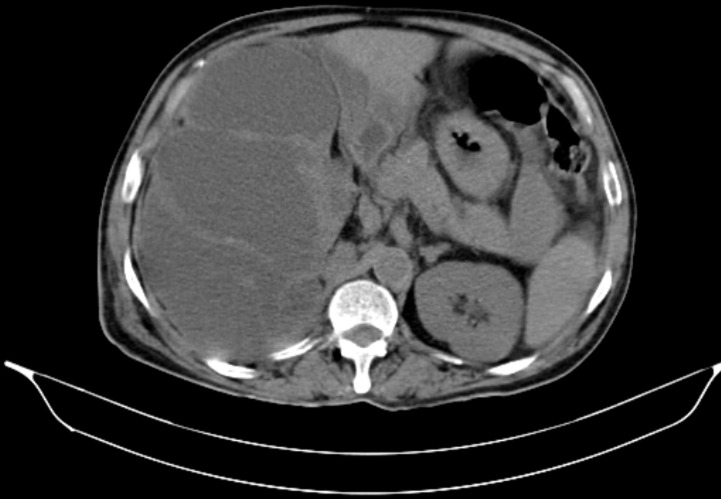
computed tomography abdomen-pelvis, large solid cystic lesion was noted in the right kidney
